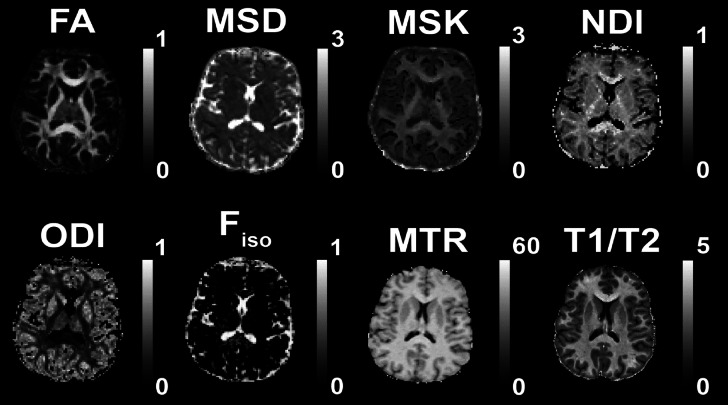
Example Brain maps of the 8 WM measures from a single subject aged 22 included in the analyses. We show a young participant’s data from 6 diffusion metrics (FA, MSD, MSK, NDI, ODI, F_iso_), plus the additional measures of MTR and T1/T2 ratio. Not shown are the raw T1w + T2w images from which total WM volume (TWM) was calculated, and the other two global measures of White Matter Hyperintensities (WMHI), which were computed across WM ROIs using the T1w and T2w images and the SAMSEG algorithm, and PSMD, which was computed across the WM ROIs using the DWI FA and MSD measures.
